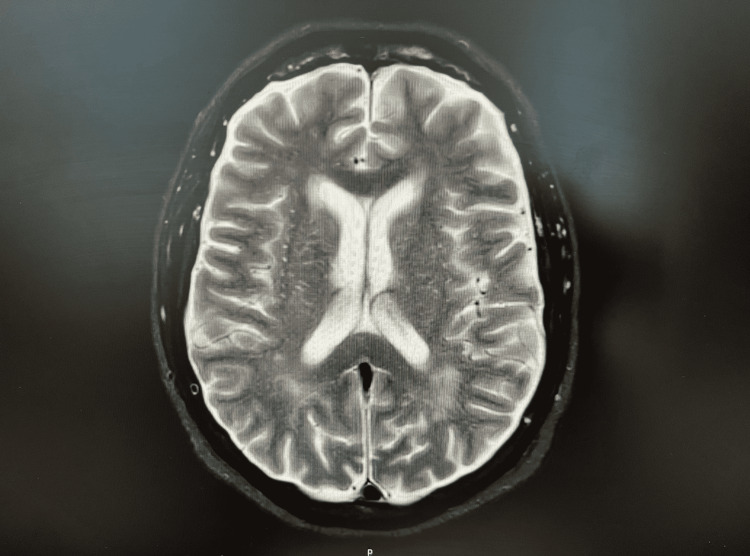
MRI of head showing dural enhancement MRI: magnetic resonance imaging
